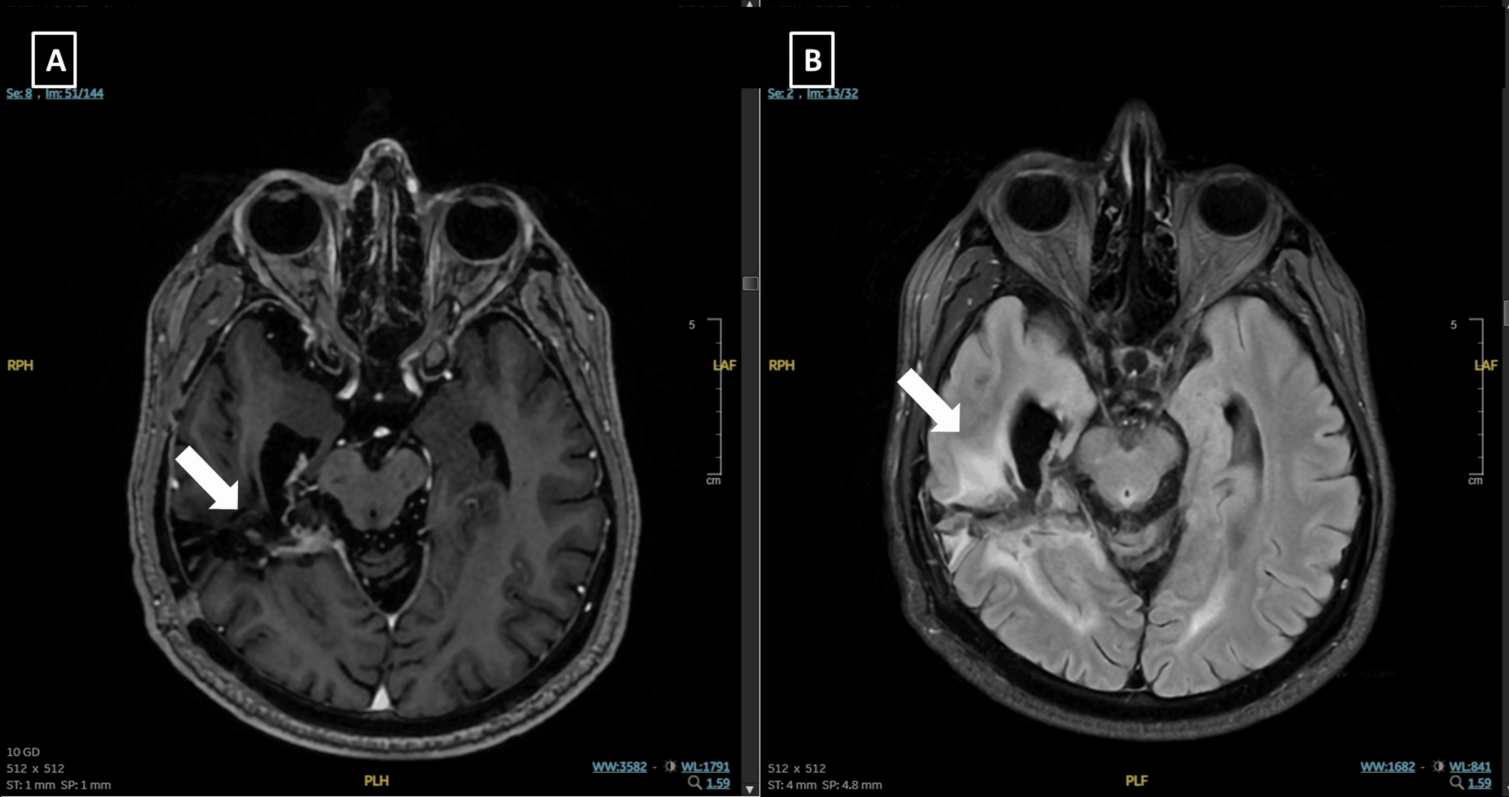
Diagnostic MRI images of Case 2. (A) Axial T1-weighted post-contrast (T1C+) MRI demonstrating a recurrence involving the left frontal and parietal lobes. (B) Axial T2 FLAIR image corresponding to the lesion.
